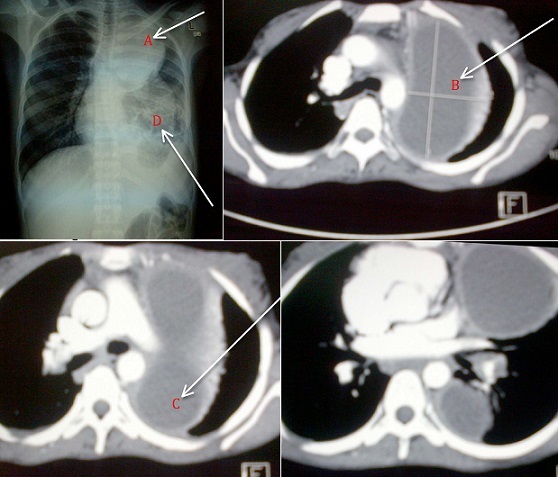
Posterior-anterior chest x-ray view showing a well circumscribed oval opacification resembling a lung mass (A), Contrasted computerized tomography (CT) of the chest showing the largest lung abscess measuring 10.75x5.93cm (B), Contrasted chest CT scan showing a lung abscess at the base of the left lung, Posterior-anterior chest x-ray view showing bronchioectatic changes in a left collapsed/fibrosed left middle lobe (D).
